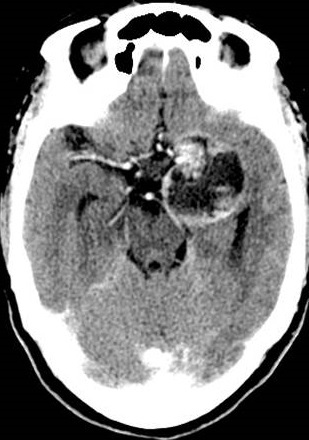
Axial Post enhanced CT scan shows left temporal lobe complex mass with peripheral thick nodular enhancement
